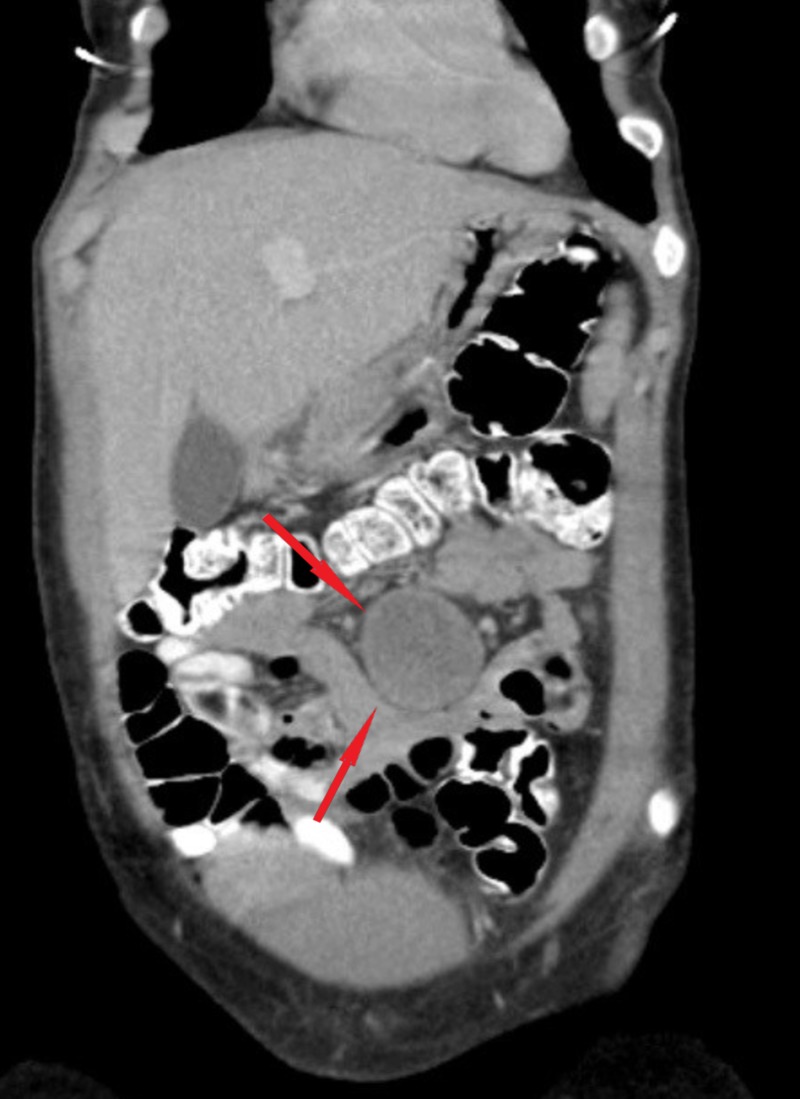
Coronal view of computed tomography scan of the abdomen showing the mesenteric cyst (red arrows)
